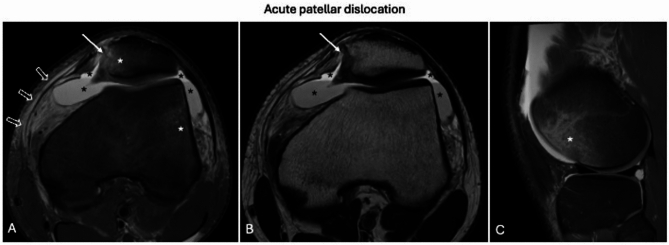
Acute patellar dislocation. Knee MRI performed 4 days after trauma shows bone edema in the inferomedial aspect of the patella (A; white asterisk) and the anterolateral aspect of the lateral condyle (C; white asterisk), with osteochondral fracture of the patella (A, B; white arrows). Also note the disruption of the medial patellofemoral ligament with peri-ligamentous edema (A; void arrows) and joint effusion with hemarthrosis (B, C; black asterisks)
